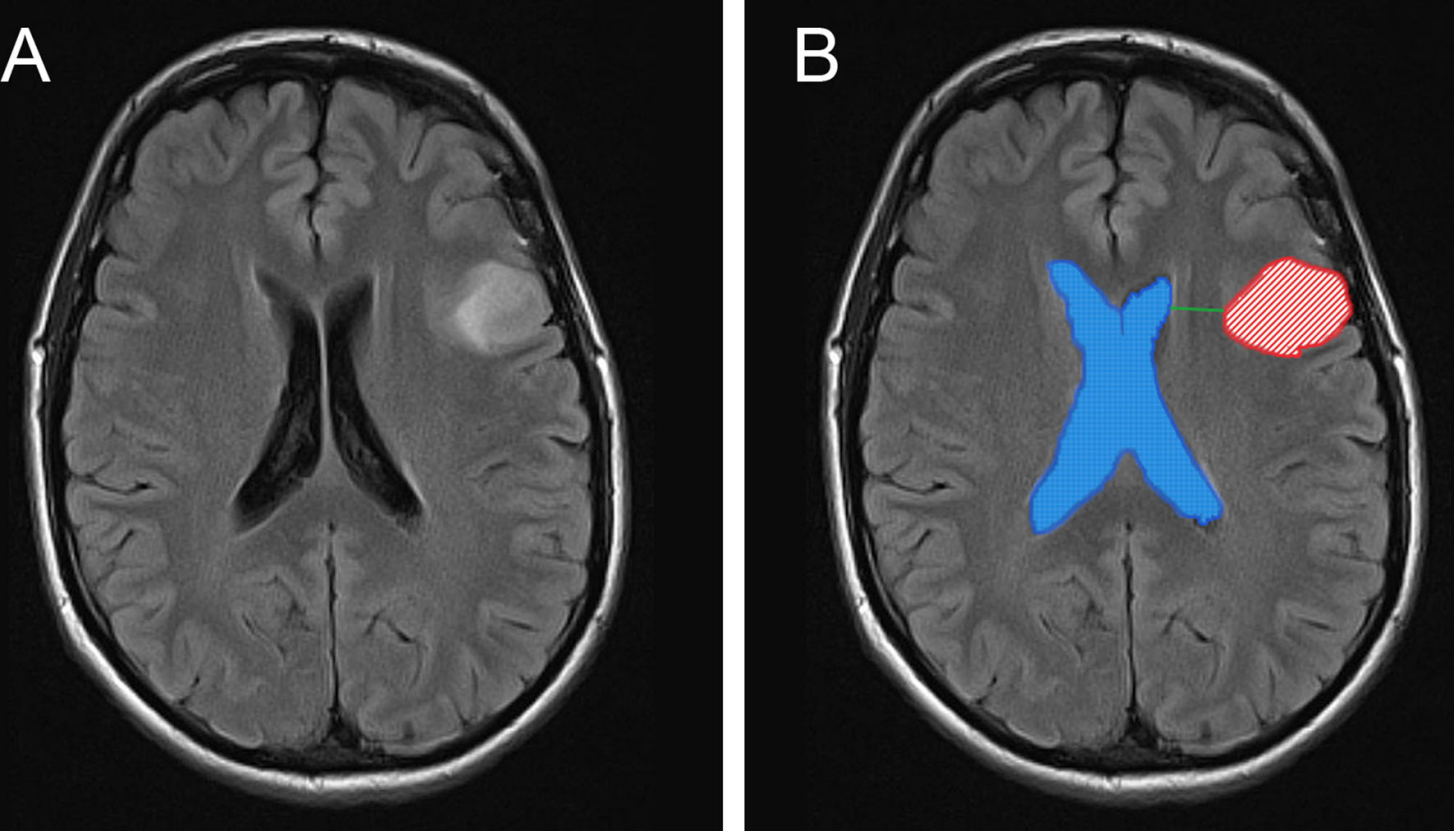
MR images depicts the anatomical position between tumors and the SVZ, calculation methods of V_G_ and V_GW_. Representative MR image of tumor without SVZ involvement (A). The tumor lesions and ventricles are manually segmented and shown as red and blue masks. The shortest distance between the tumor edge and the lateral ventricles was showed as green line (B).
